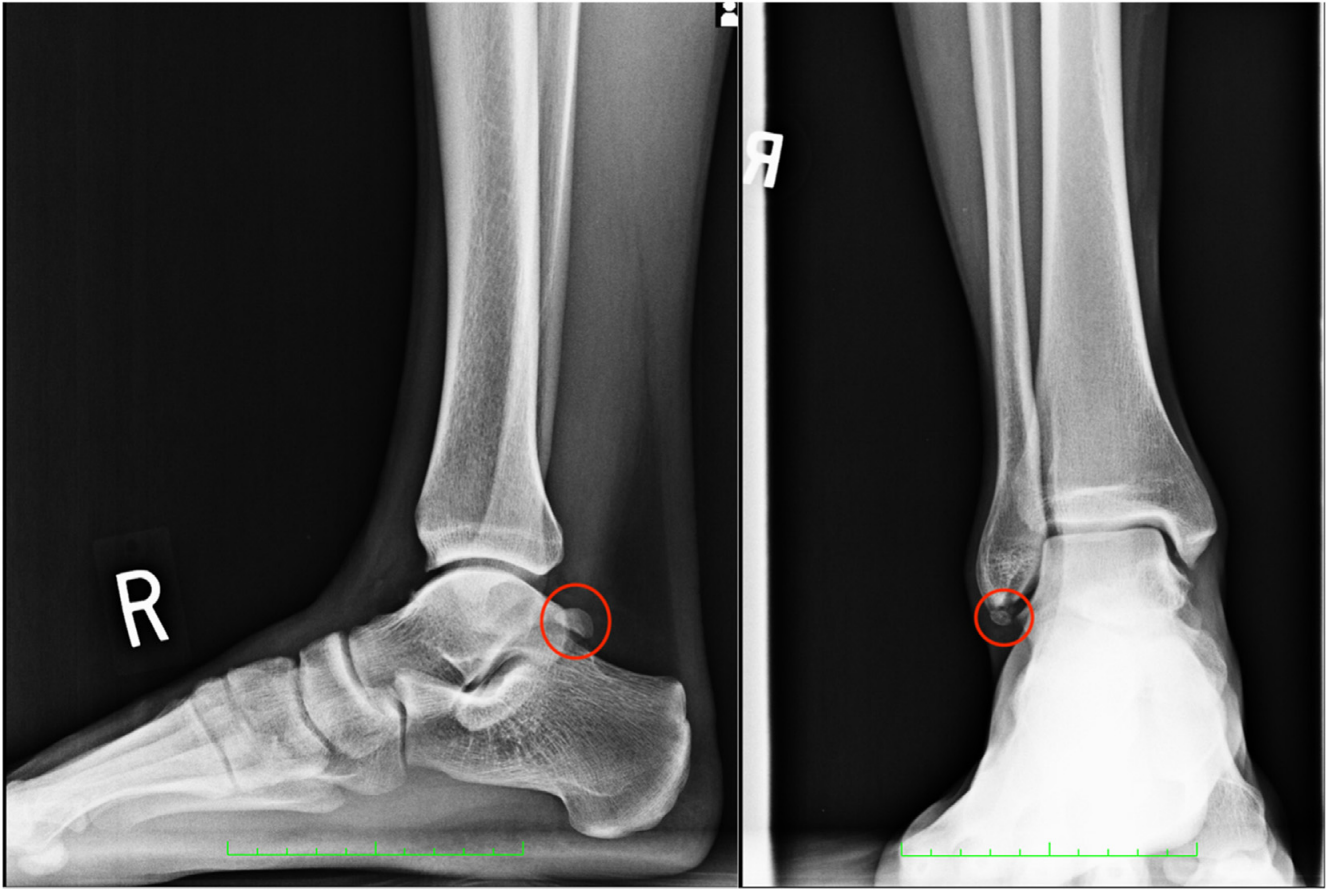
Os trigonum (left) and os subfibulare (right). The left image is a right ankle, lateral radiograph. The right image is a right ankle, anteroposterior radiograph.
